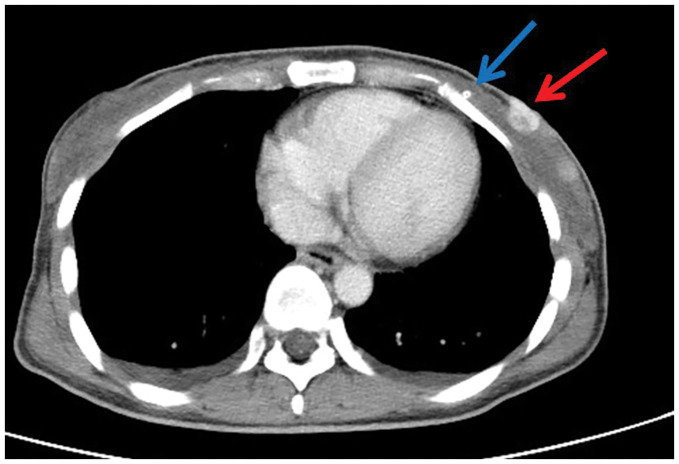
Contrast-enhanced CT of the chest and abdomen. A round, 20-mm mass with contrast enhancement was observed in the medial region adjacent to the nipple along with a hyperdense structure thought to be the catheter. (Blue arrow: catheter; red arrow: mass.)
